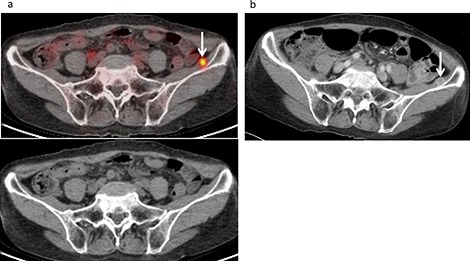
Muscle metastasis developed in a 73-year-old female following treatment for malignant pleural mesothelioma, including neoadjuvant chemotherapy, pleurectomy and decortication procedures, and chemotherapy. (a) FDG-PET/CT and CT findings revealed moderate FDG uptake (SUVmax 4.2) in the left iliac muscle (arrow), suggesting muscle metastasis with a score of 4. (b) Contrast-enhanced CT revealed an enhanced mass (10 mm) in the left iliac muscle (arrow), which had not been detected by either of the examiners (false negative).
